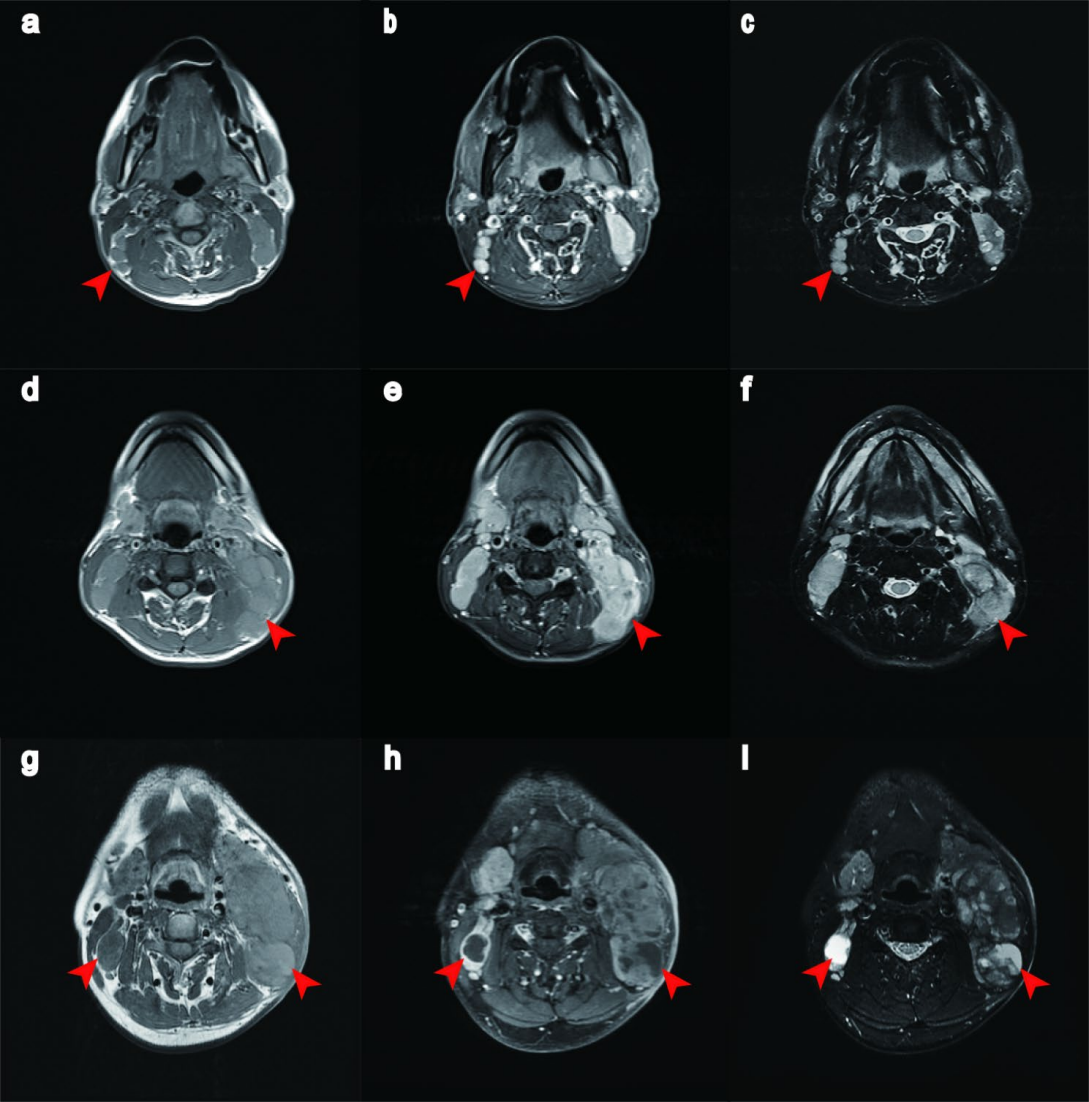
Representative MR images of different lymph node features (a) Axial T1-weighted, (b) axial contrast-enhanced T1-weighted, and (c) axial T2-weighted images in an NPC patient with NG (arrows). (d) Axial T1-weighted, (e) axial contrast-enhanced T1-weighted, (f) axial T2-weighted images in a patient with ENE (arrows). (g) Axial T1-weighted, (h) axial contrast-enhanced T1-weighted, (i) axial T2-weighted images in a patient with LNN (arrows)
